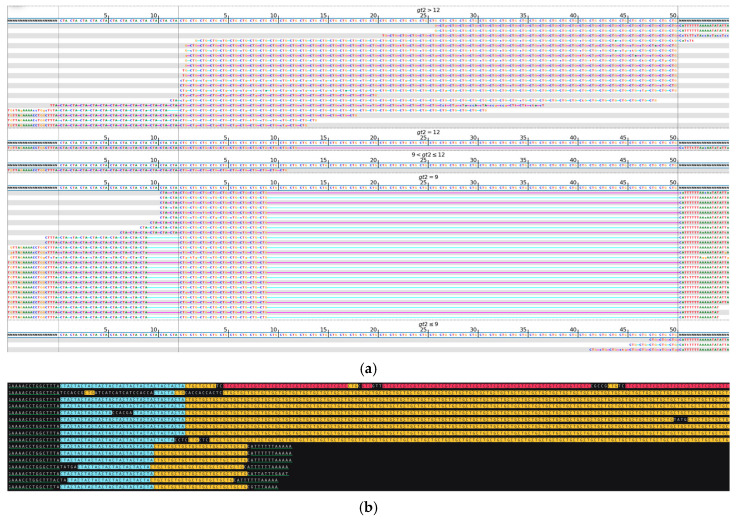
ATXN8OS*, heterozygous repeat expansion, complex region with CTA-CTG structure. (a) Visualization of short-read sequencing results showing flanking the STR regions, as well as those and reads fully contained within the repeat. The bioinformatically predicted genotype based on the read statistics is 9 + 9/12 + 79 * repeats (CAT + CTG/CTA + CTG complex region). (b) Visualization of the ONT long-reads sequencing results showing total expansion length and structure—9 CAT + 9 CTG repeats (shorter, normal allele) and 12 CTA + 83–84 CTG repeats (expanded allele). Green-underlined sequences indicate regions flanking the STR repeat. Different repeat units are highlighted in different colors: CTG (yellow), CTA (blue), GTC (red). * Results of bioinformatic prediction not depicted in the image.
